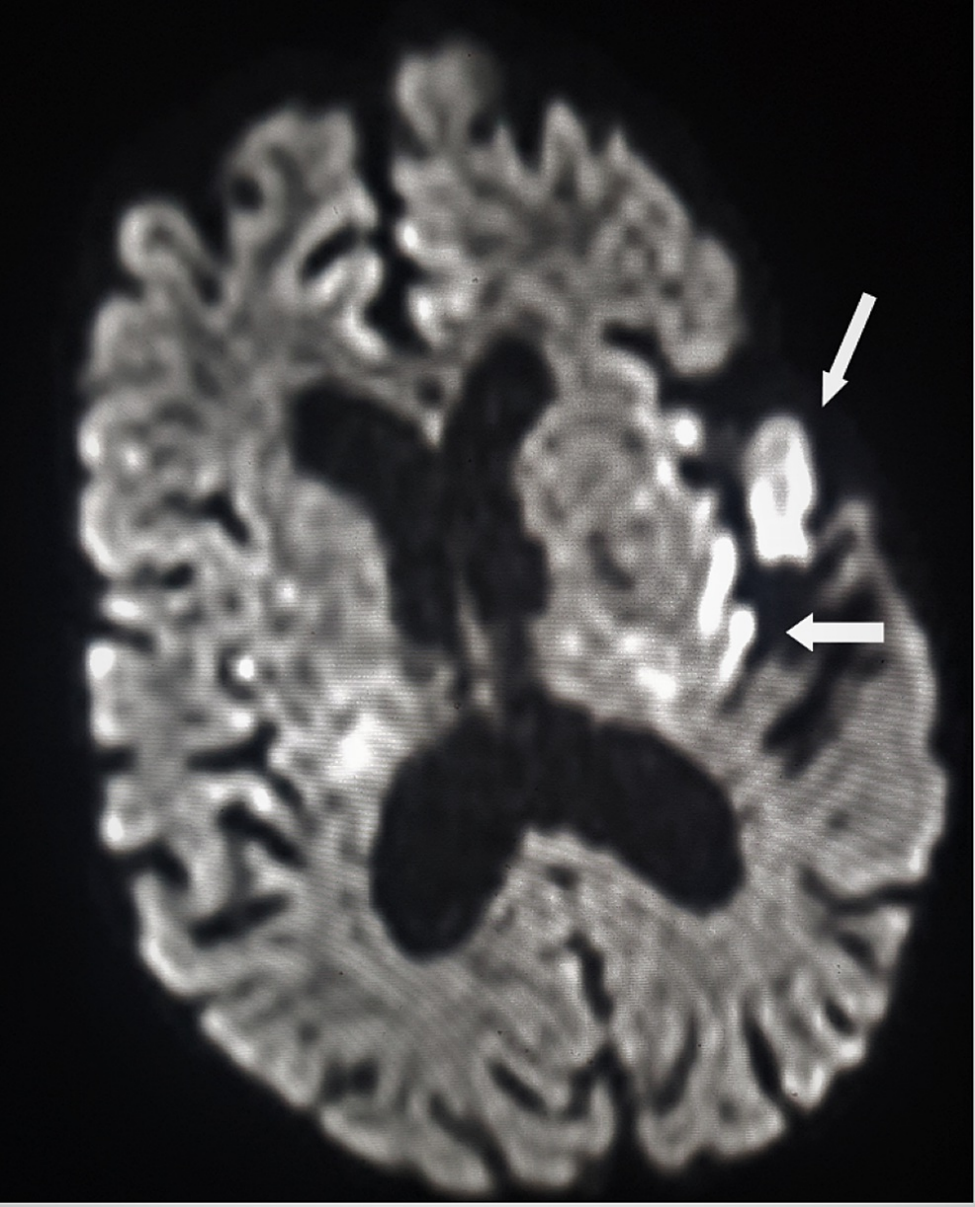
MRI brain with focal areas of hyperintense signal on DWI with signal drop seen in the left frontal periventricular region and left insular cortex, suggesting acute infarct (white arrow) DWI: diffusion-weighted imaging
